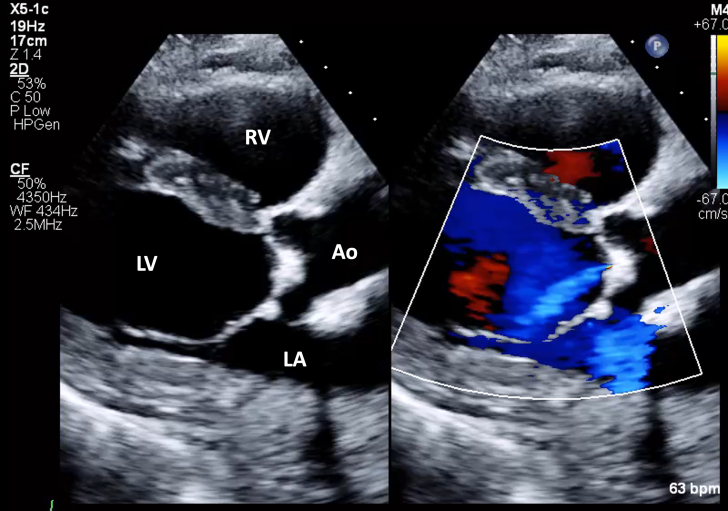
Zoomed Parasternal Long-Axis View With and Without Color Doppler Zoomed in image of the aortic valve in parasternal long-axis view with and without color Doppler. Abbreviations as in Figure 2.
